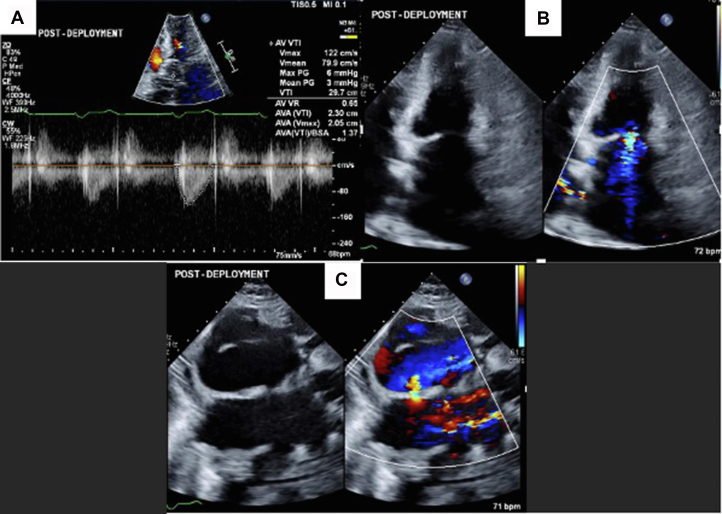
Postoperative Echocardiography Postoperative transthoracic echocardiography showing normal function of the prosthetic aortic valve (A), mild mitral regurgitation (B), and a small atrial septal defect with left to right shunting (C).
